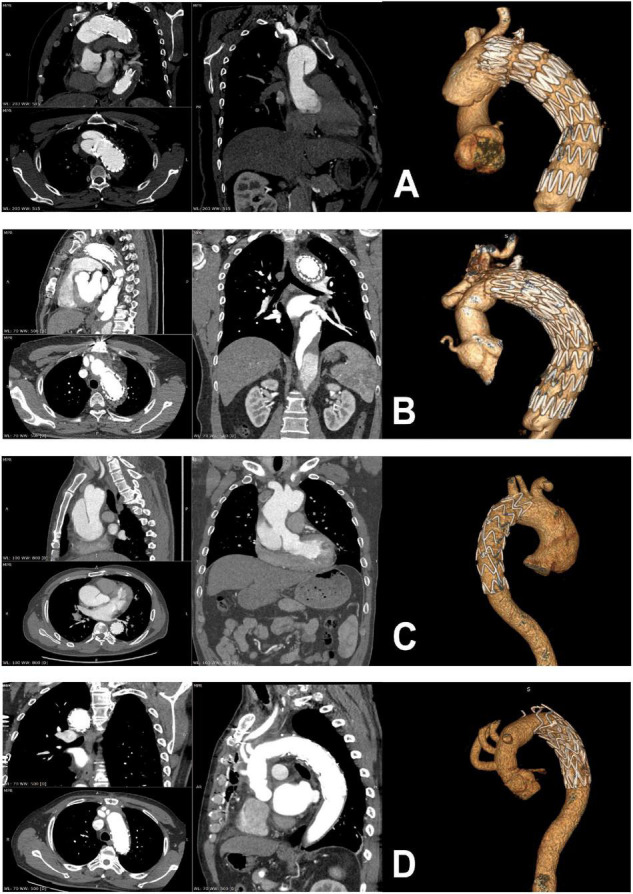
Preoperative and postoperative computed tomographic scans of patients with retrograde type A dissection (A,B) and antegrade type A dissection (C,D).
